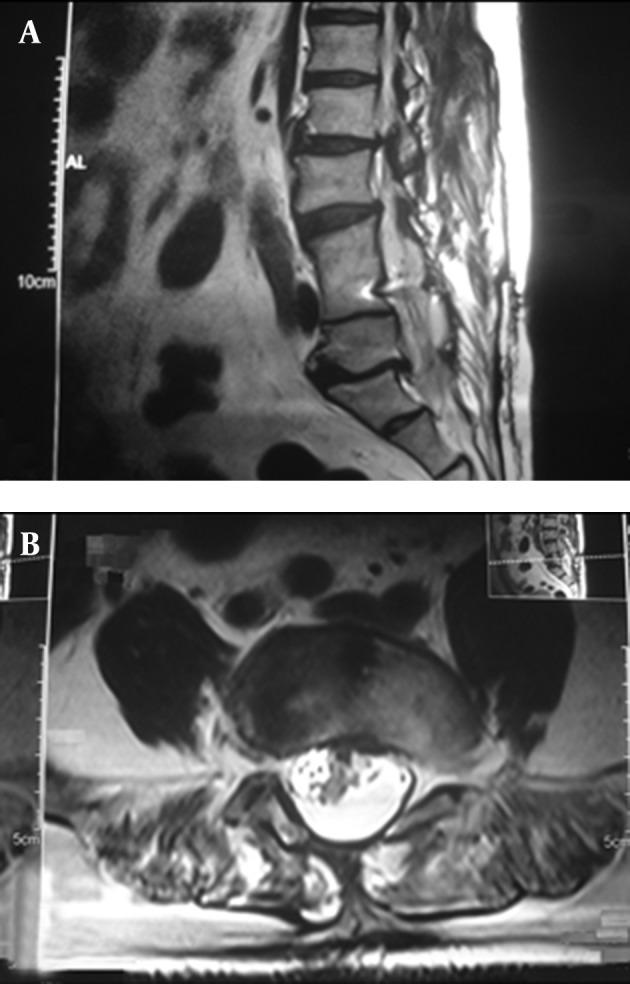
Mid-sagittal (A) and Axial (B) T2-weighted MRI Images Showing Removal of the Bony Spur and Release of the Spinal Cord
